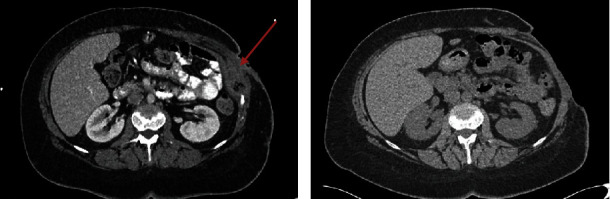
Computed tomography of abdomen (a) pre- and (b) postoperative. (a) Arrow marks the abscess with trapped air. (b) Postoperative lesion of the left abdominal wall.
